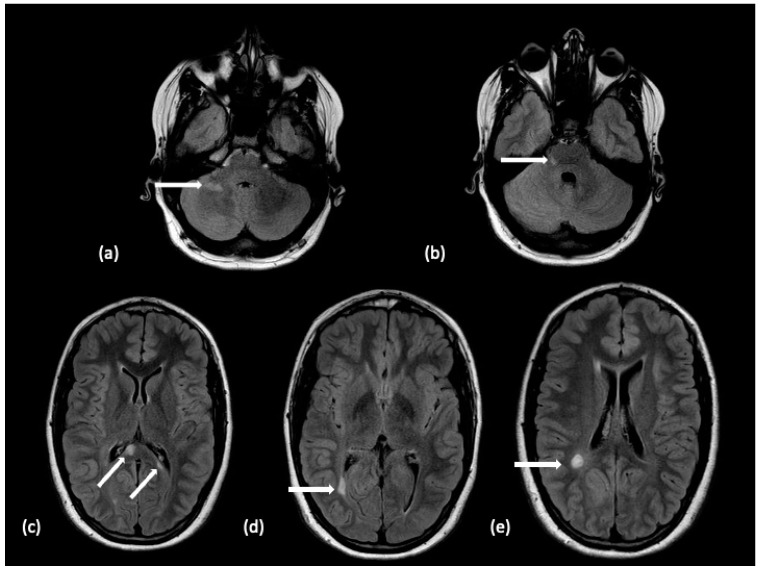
A 14-year-old male with a 3-year history of multiple demyelinating, non-enhancing brain lesions. A follow-up MRI demonstrated an increase in both size and number of lesions with a distribution within the brain characteristic of pediatric-onset multiple sclerosis. Non-enhancing lesions on an axial FLAIR sequence without diffusion restriction are noted with white arrows within the (a) right hemisphere cerebellar white matter, (b) dorsal right pons, (c) splenium of the corpus callosum, (d) bordering the right occipital horn, and (e) right hemispheric parietal white matter. The features supported the diagnosis of pediatric-onset multiple sclerosis.
